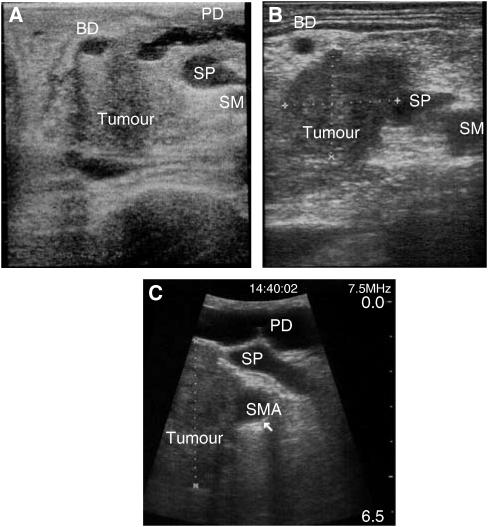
Laparoscopic ultrasound assessment of vascular invasion. (A) Resectable tumour free from splenoportal junction. (B) Tumour involvement of splenoportal junction. (C) Tumour involvement of the superior mesenteric artery (BD – bile duct, PD – pancreatic duct, SP – splenoportal junction, SMA – superior mesenteric artery).
